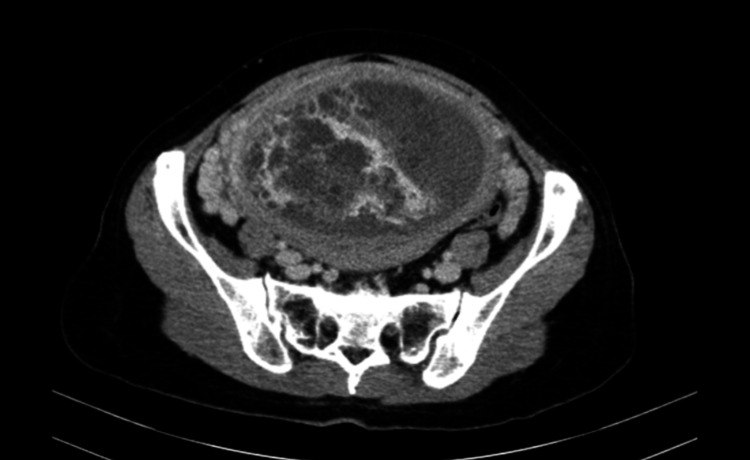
Contrast-enhanced CT appearance of the hydatidiform mole
